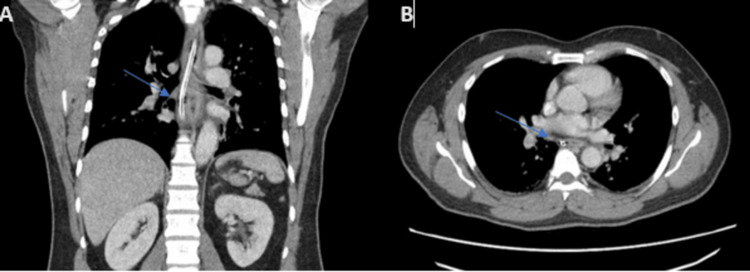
(A) Axial section CT image showing a nasogastric tube passed under the endoscopic vision across the perforated area at the level of thoracic vertebra. (B) Coronal section CT image with contrast showing a small linear contained leak draining into a small walled-off collection measuring approximately 24 x 9 mm, to the left of esophagus (arrow).
